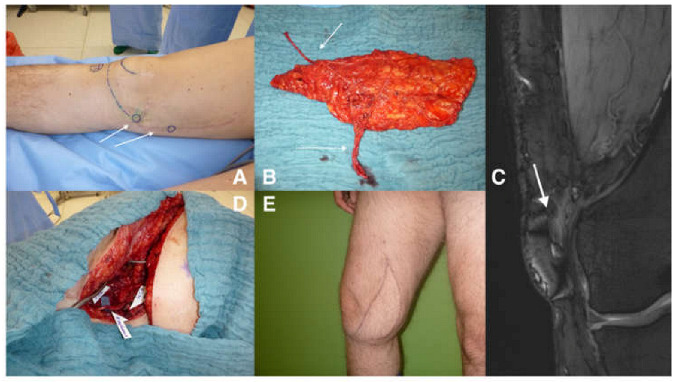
Clinical demonstration of the procedure. (A) shows the areas with positive Hoffmann–Tinel sign after arthroscopy of the knee. The neuroma formation was also detected in MRI scans (C). (B) shows the cutaneous branch beside the vascular pedicle, which was anastomosed to the femoral artery and greater saphenous vein (D), as well as coaptated with the remaining saphenous nerve. Final result is not only functional but also cosmetically appealing for the patient (E).
